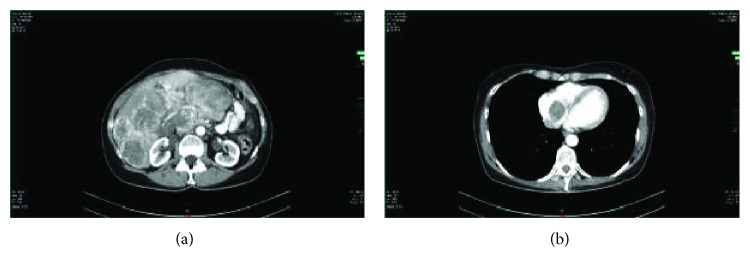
(a) Abdominal CT scan with IV contrast showing multiple liver metastases. (b) Chest CT scan showing a filling defect within the contrast-enhanced right atrium which was initially thought to be a large thrombus.
